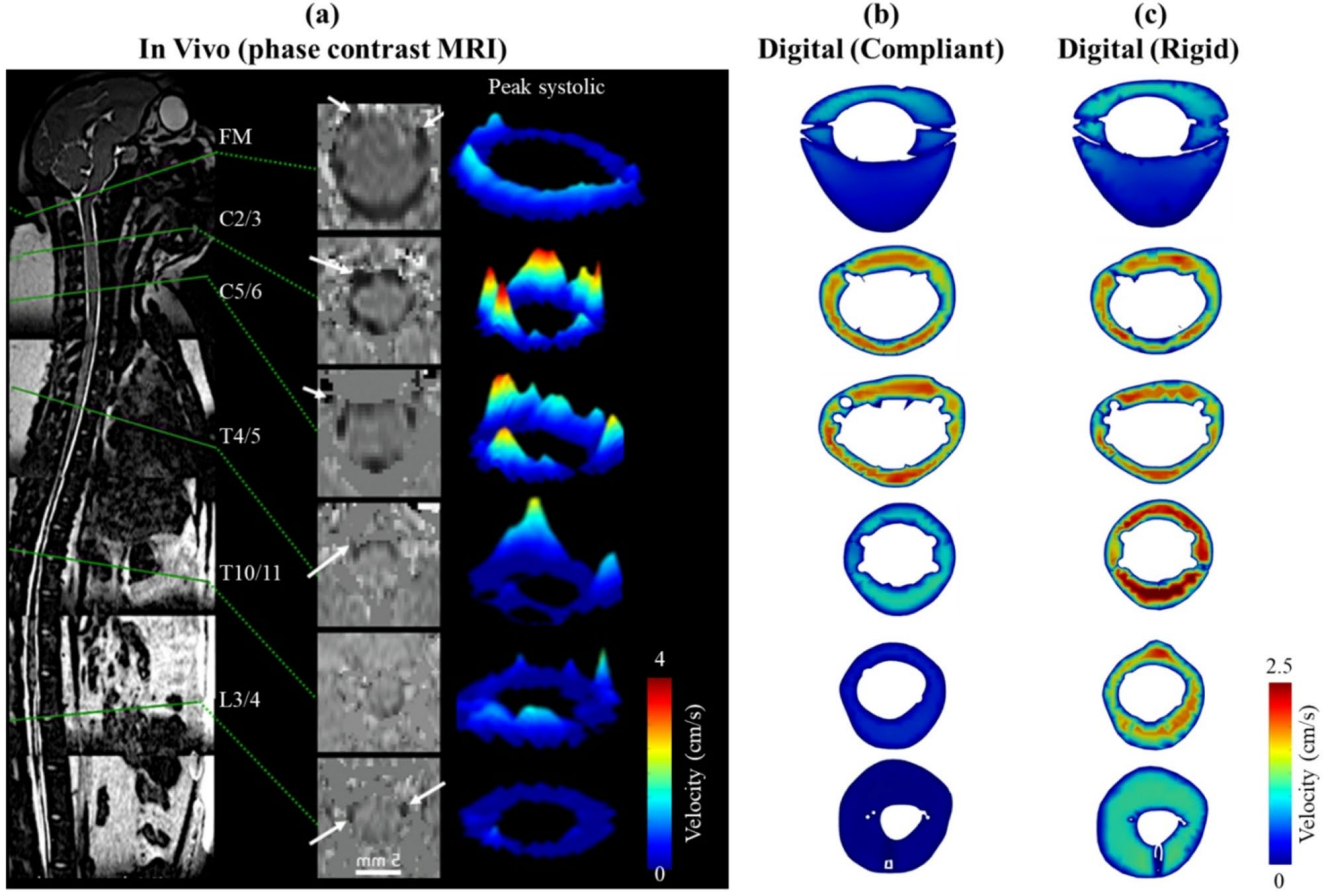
Agreement of in vivo MRI velocity field compared to the compliant and rigid digital model. (a) In vivo depiction of NHP T2-weighted MRI mid-sagittal view with axial phase contrast MRI peak CSF velocity distribution around the spinal cord and 3D velocity field visualization (image modified from [30]), (b) Compliant digital model velocity profile at foramen magnum, C2/3, C5/6, T4/5, T10/11 and L3/4 vertebral levels, and (c) Rigid digital model velocity profiles at same locations
